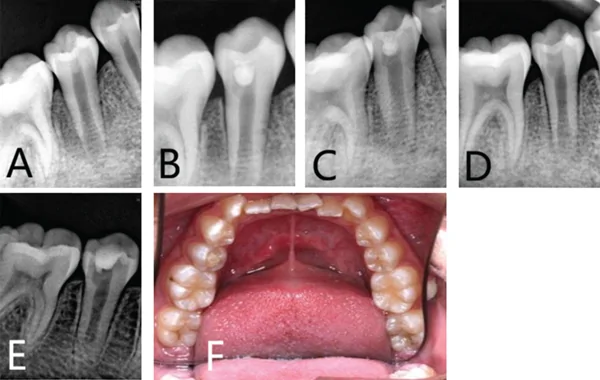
(A) Preoperative periapical radiograph; (B) 2‐month postoperative radiograph; (C) 9‐month postoperative radiograph; (D) 10‐month postoperative radiograph; (E) 27‐month follow‐up periapical radiograph; and (F) intraoral photograph at the 27‐month follow‐up.
